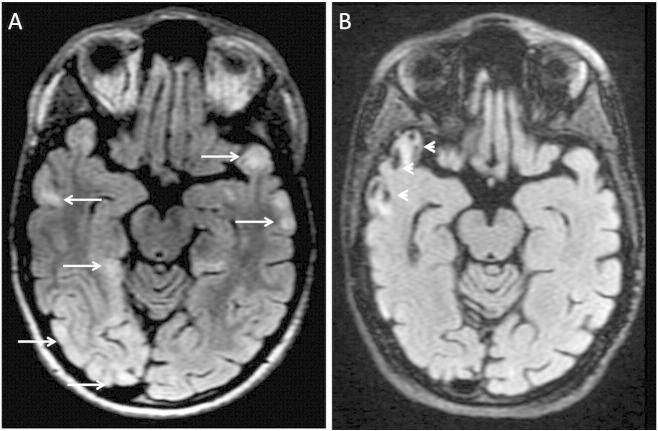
Axial FLAIR (Fluid Attenuation Inversion Recovery, figure A) sequence shows multiple areas of brain parenchymal high signal (long white arrow) in the temporal lobes bilaterally and the right occipital lobe. FLAIR image 4 years later (figure B) reveals an old infarct in the right temporal lobe (white arrowheads) but the other areas of signal abnormalities have returned to normal.
